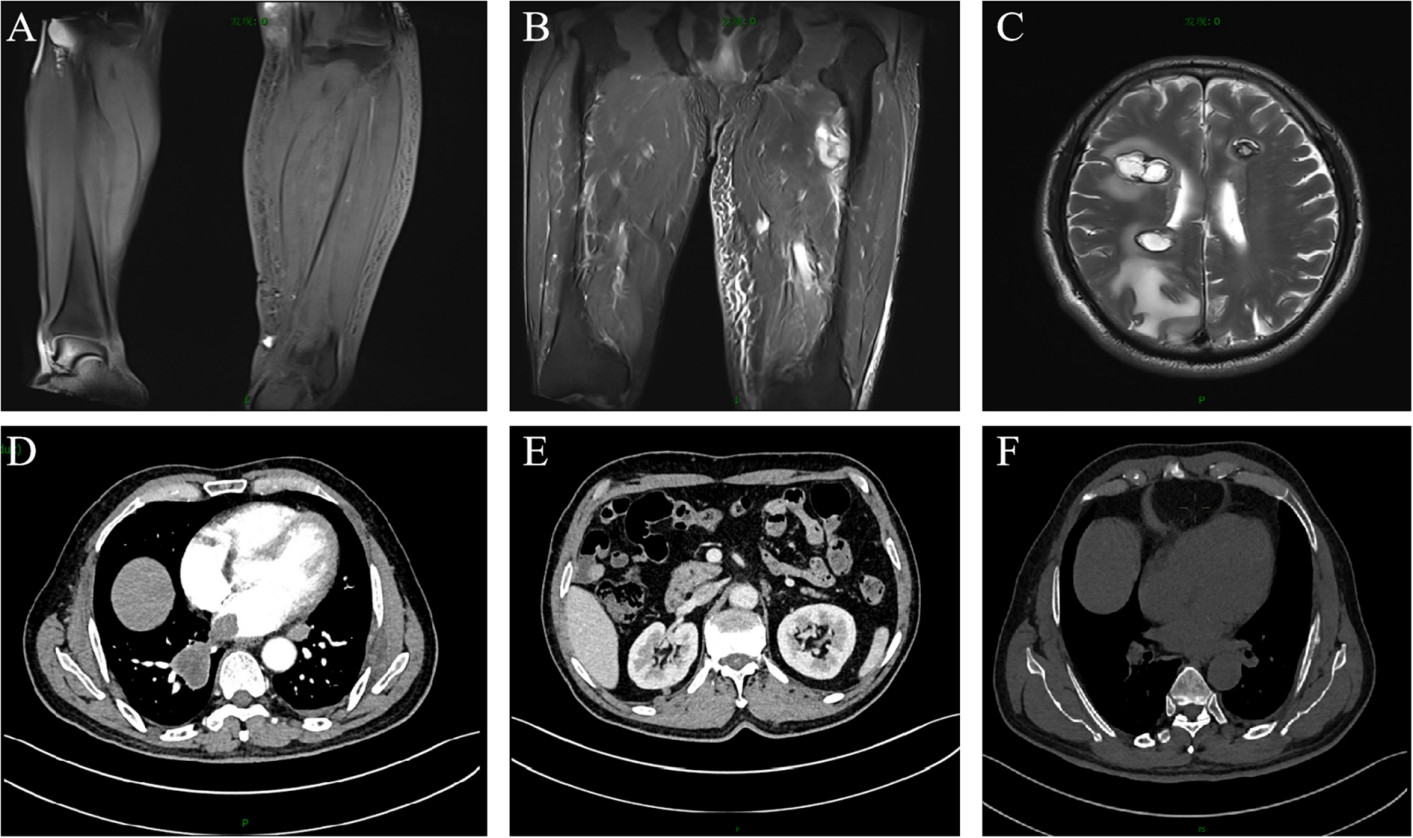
Enhanced magnetic resonance imaging and computed tomography scans of the patient. Metastatic lesions in the: (A) left calf; (B) left thigh; (C) brain; (D) lungs and pericardium; (E) kidneys; (F) bones.
